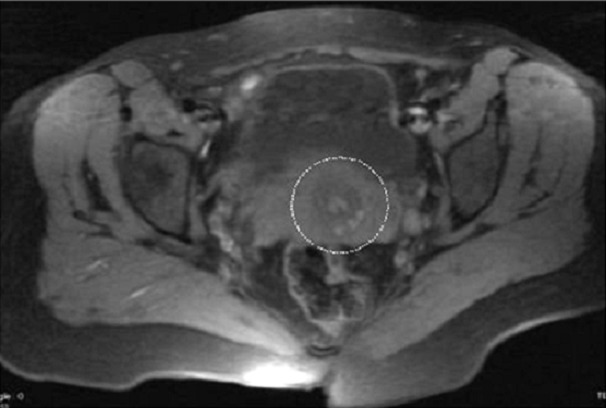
Pelvic MRI: axial sequence (T1 FATSAT) evidenced multiple round lesions in hypersignal T1 facing the cervical vaginal area
